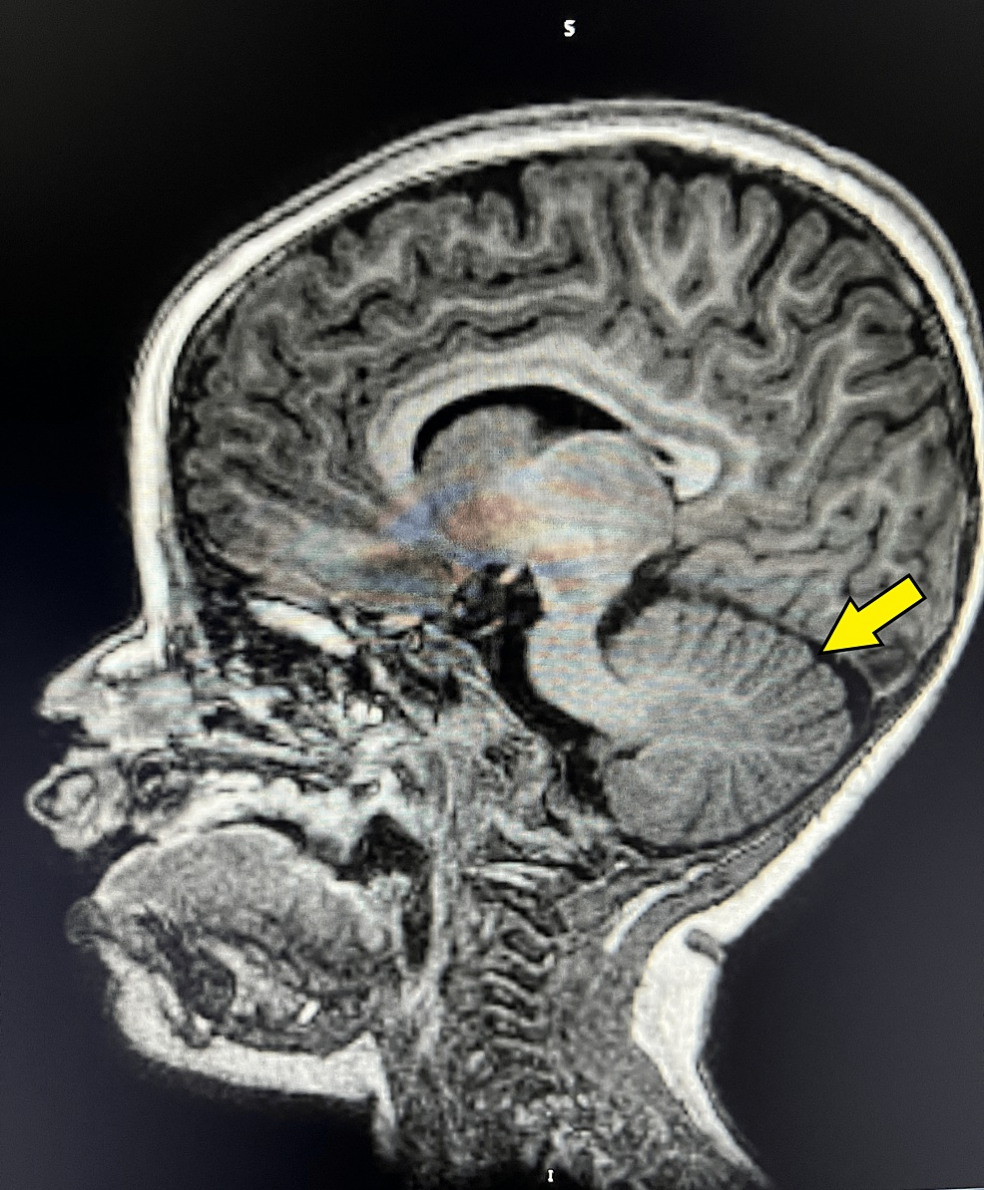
T1-weighted sagittal MRI A T1-weighted image displays a serrated pattern and cortical thickening in the peripheral cerebellar folia (yellow arrow).
